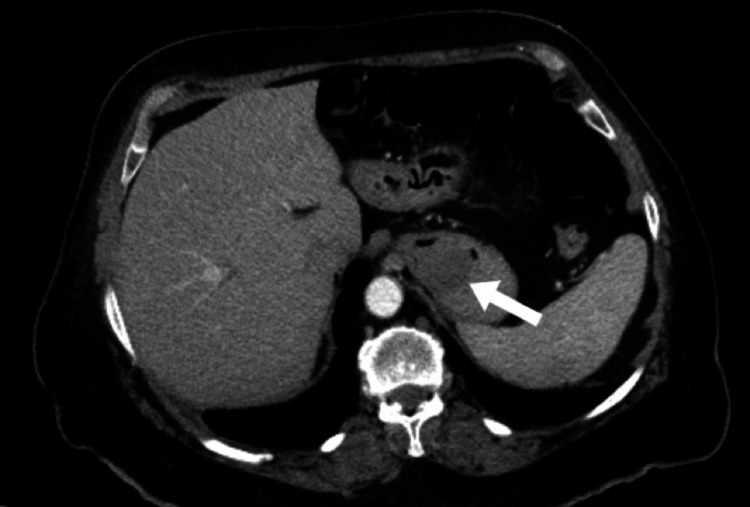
Computed tomography with contrast in axial plane. Gastric leiomyoma is indicated by the arrow.
